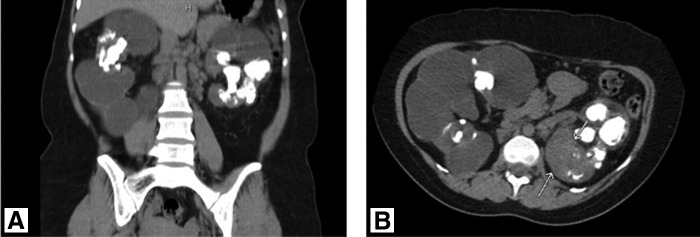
Case 2: Original preoperative CT scan from June 2015 (A: coronal, B: axial) demonstrating bilateral staghorn calculi and a hyperdensity in the central region of the left kidney (*arrows*) felt to represent indeterminate, possibly representing hemorrhagic or proteinaceous debris.
